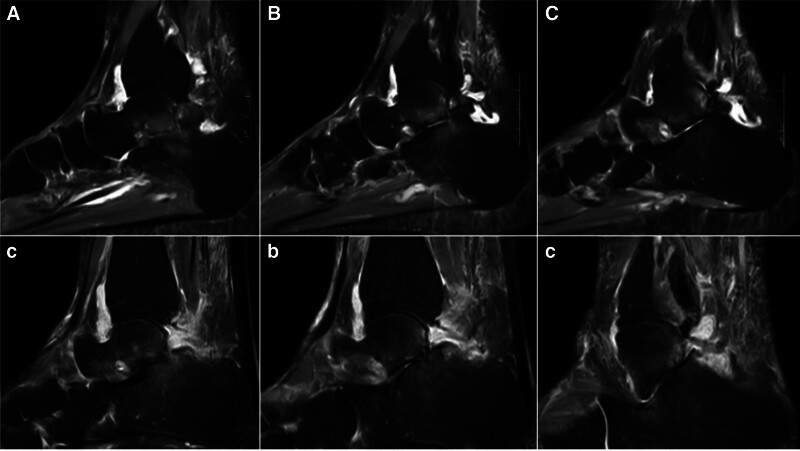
(A–C) Preoperative sagittal MRI scan images show relatively severe bone marrow edema signals in the anterior ankle joint and posterior talus, and relatively mild bone marrow edema signals in the subtalar joint. (a–c) Postoperative sagittal MRI scan images shows that the bipartite talus has been almost completely resected. CT = computed tomography, MRI = magnetic resonance imaging.
